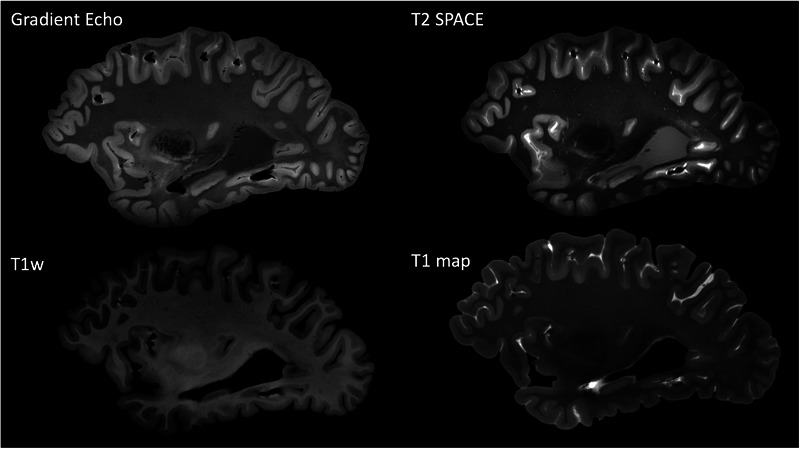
Example of acquired MR contrasts for postmortem brain MRI. T2 SPACE, T2 sampling perfection with application‐optimized contrast using different flip angle evolution; T1w, T1‐weighted image from magnetization‐prepared two rapid gradient echo (MP2RAGE) sequence; T1 map, quantitative T1 relaxation time map from MP2RAGE acquisition.
